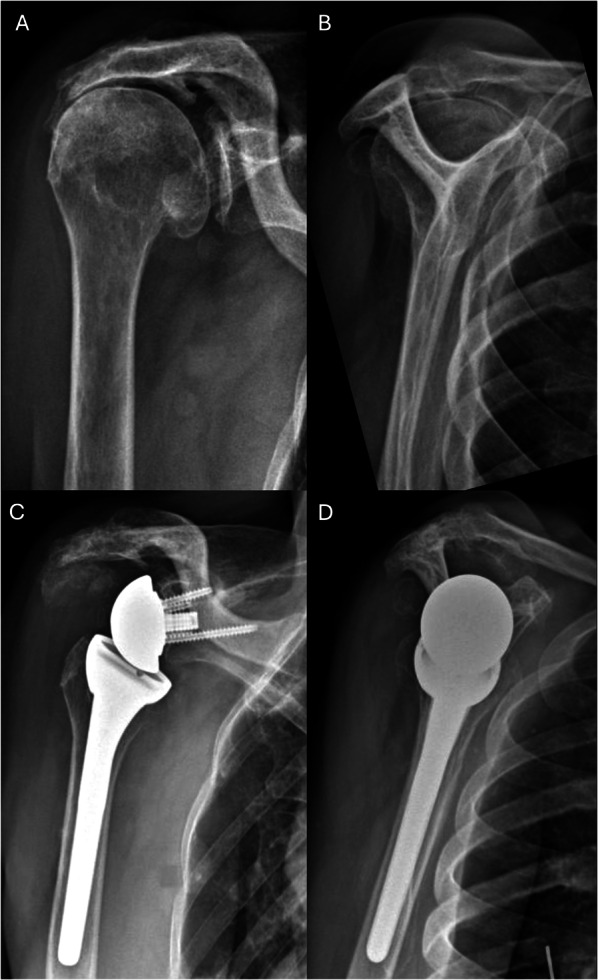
Clinical case of a 73-year-old female patient with cuff tear arthropathy and combined loss of elevation and external rotation (CLEER) undergoing reverse shoulder arthroplasty with concomitant latissimus dorsi transfer. A, B Preoperative anteroposterior and Y-view radiographs of a right shoulder. C, D Postoperative anteroposterior and Y-view radiographs
